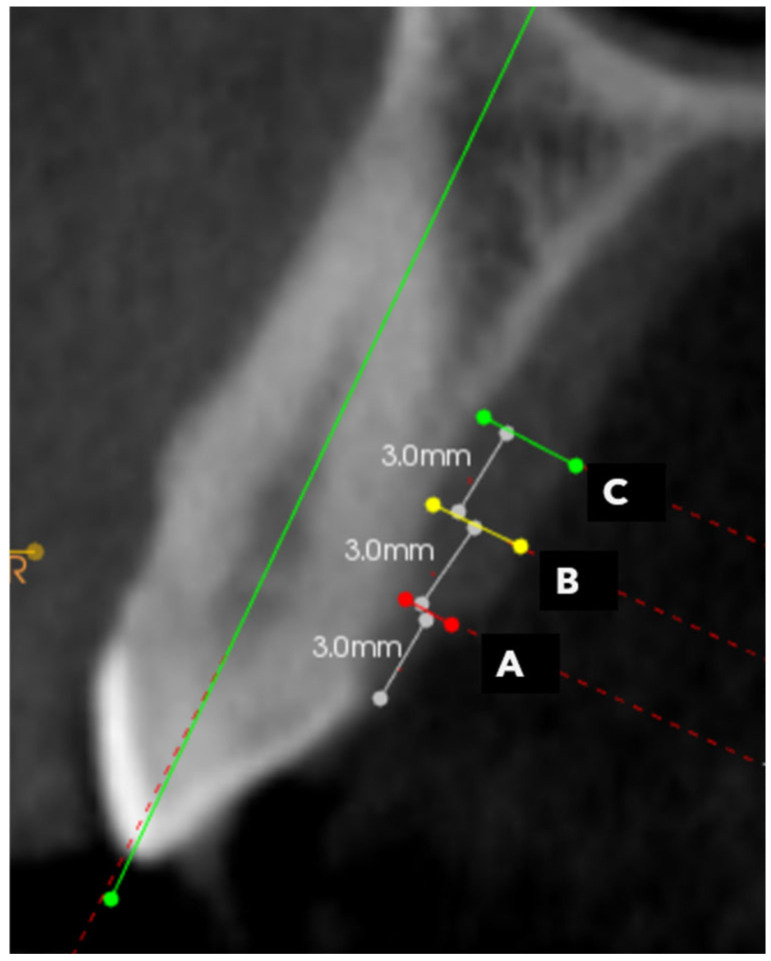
CBCT image of the measurement sites (A: 3 mm, B: 6 mm, C: 9 mm from CEJ).
